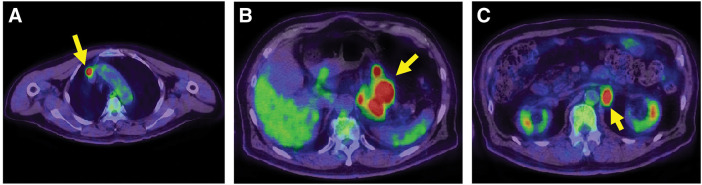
FDG-positron emission-tomography-computed tomography. (A) High FDG uptake in the anterior mediastinal lymph node was observed (SUVmax = 16.48) (arrow). (B) High FDG uptake in the primary tumor and surrounding lymph nodes was observed (SUVmax = 13.15) (arrow). (C) High FDG uptake in the para-aortic lymph node was observed (SUVmax = 9.96) (arrow). FDG, ^18^F-fluorodeoxyglucose; SUVmax, maximum standardized uptake value
